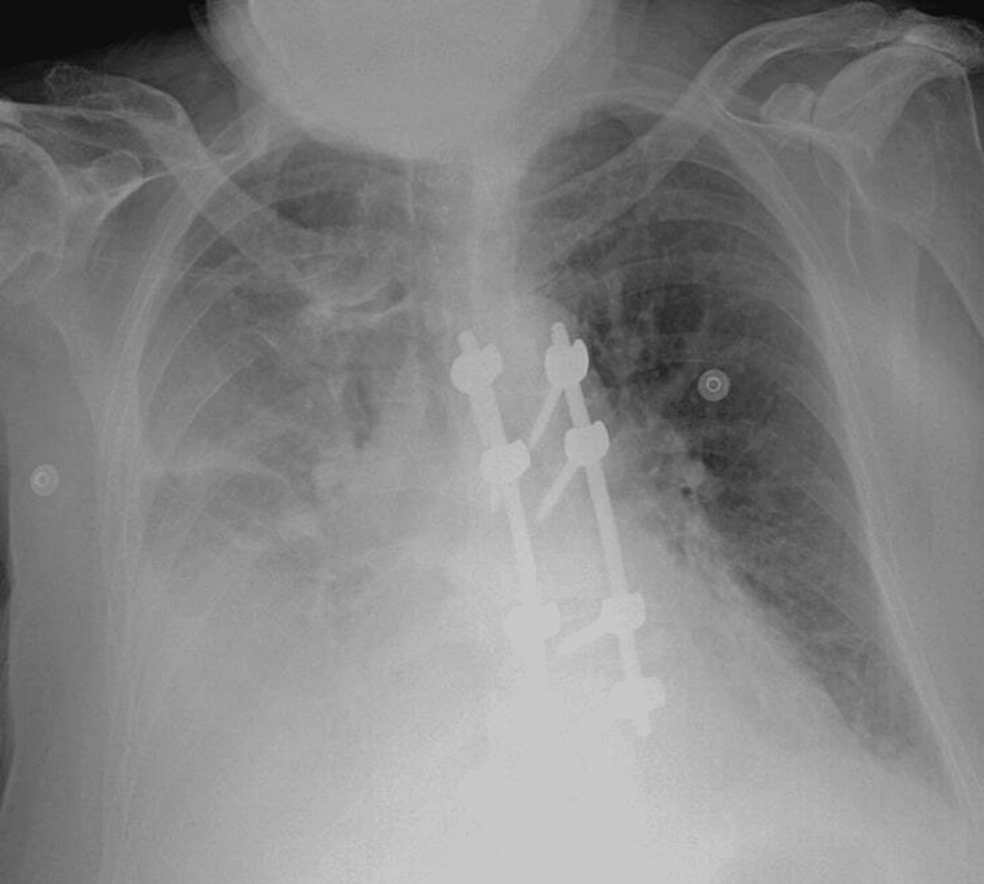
X-ray chest indicating a pleural effusion on the right side. The figure was generated entirely for this publication and gained agreement from the patient to post it.
